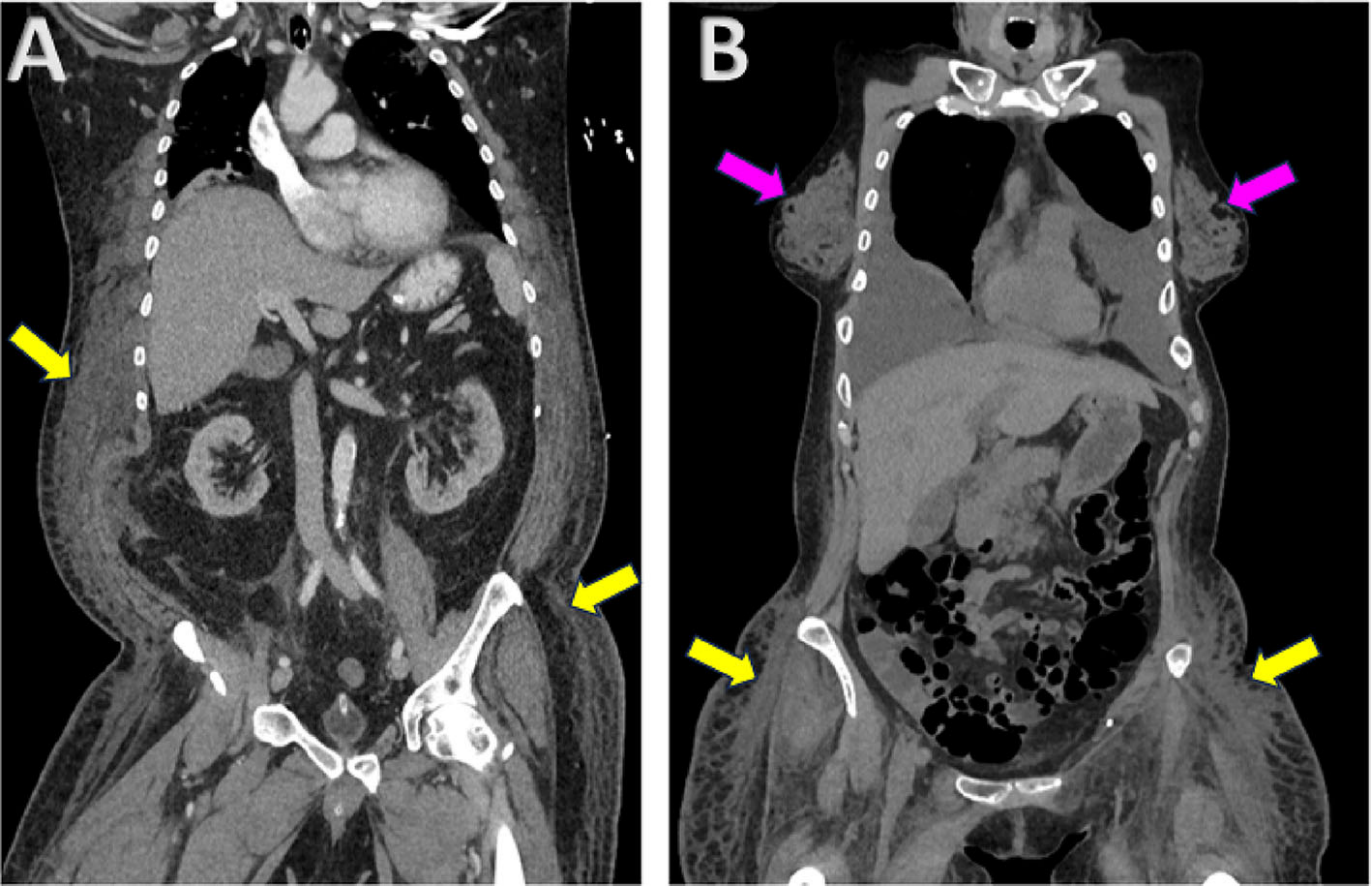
CT scans of male (A) and female patients (B), with edema present throughout the subcutaneous adipose tissue (yellow arrows). While edema has visible intensity differences with surrounding adipose tissue, it has complex shapes, unclear boundaries, and similar intensity characteristics with other tissues, e.g., glandular breast tissue in women (magenta arrows)
